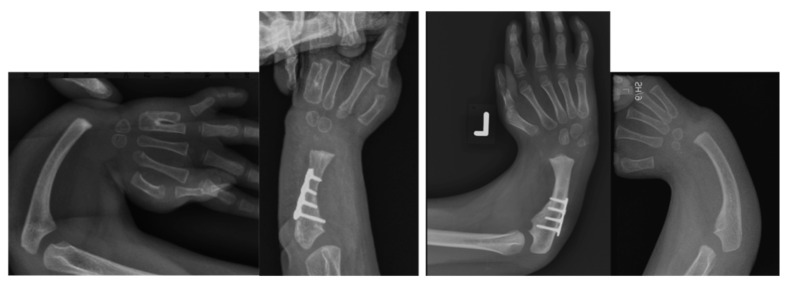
Case 9 left, 10 right. Bilateral RCH preop radiographs (left most, right most). Postop radiographs after ulnarization G3 of both hands (left middle, right middle). Note the hypertrophy of the head of the ulna after surgery in both hands.
